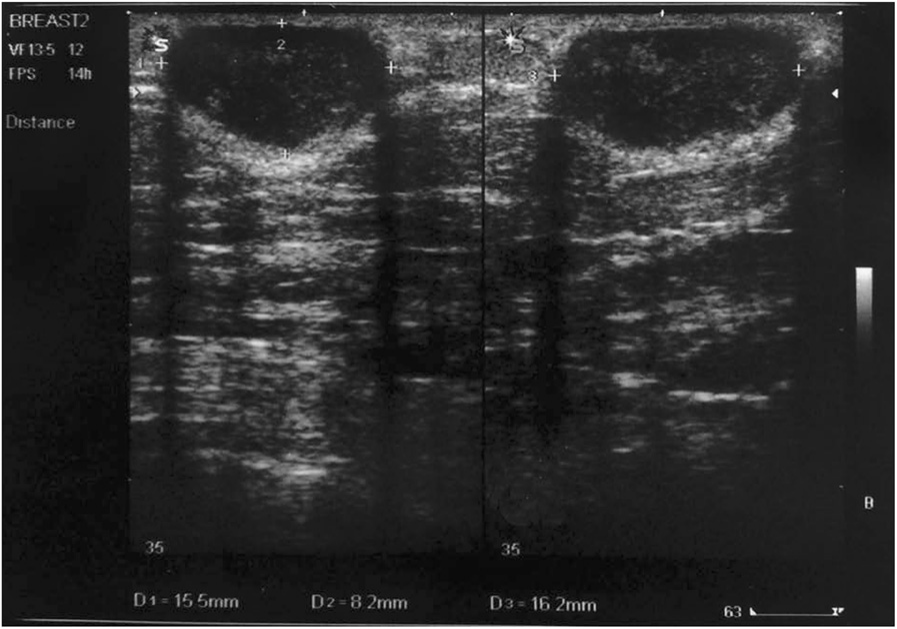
Ultrasonographic image demonstrating a well-defined hypoechoic ovoid mass with low amplitude internal echoes and reflective shadowing at the edges.
